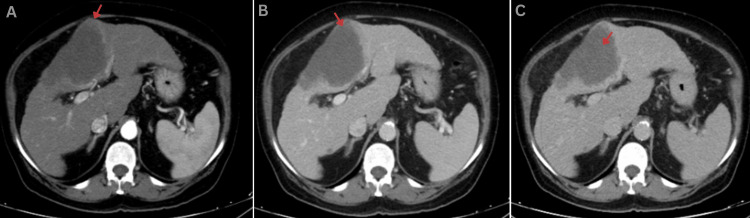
Axial contrast-enhanced CT of the abdomen. Panel A (arterial phase) demonstrates a large, lobulated, heterogeneously enhancing mass occupying segment IV of the liver, showing peripheral rim enhancement. Panel B (venous phase) shows persistent heterogeneous enhancement without washout, with poorly defined infiltrative margins. Panel C (delayed phase) reveals irregular progressive centripetal fill-in enhancement within the lesion.
